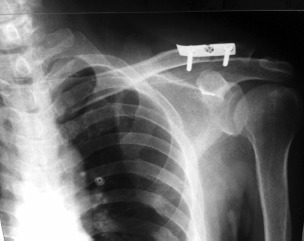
Radiograph showing reduced acromioclavicular joint. TightRope was supplemented with one-third tubular plate as additional anchor.
